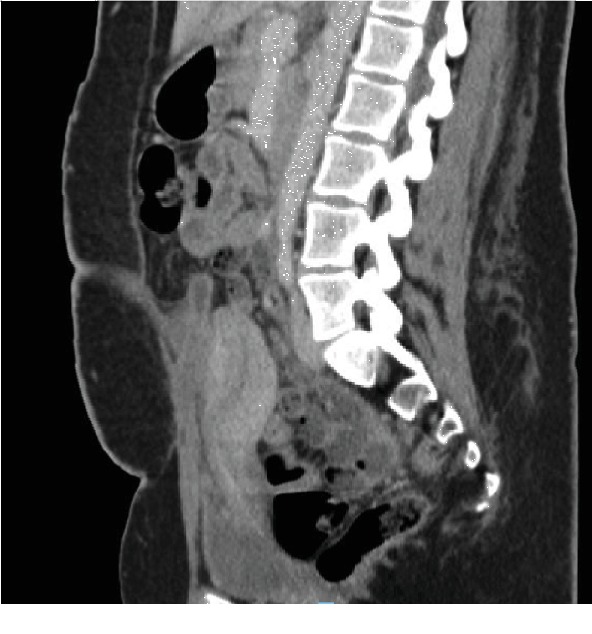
Follow-up CT scan showing reduction of the abdominal fluid collection after drainage and antibiotics 3 months after drainage (4 months postoperatively).
